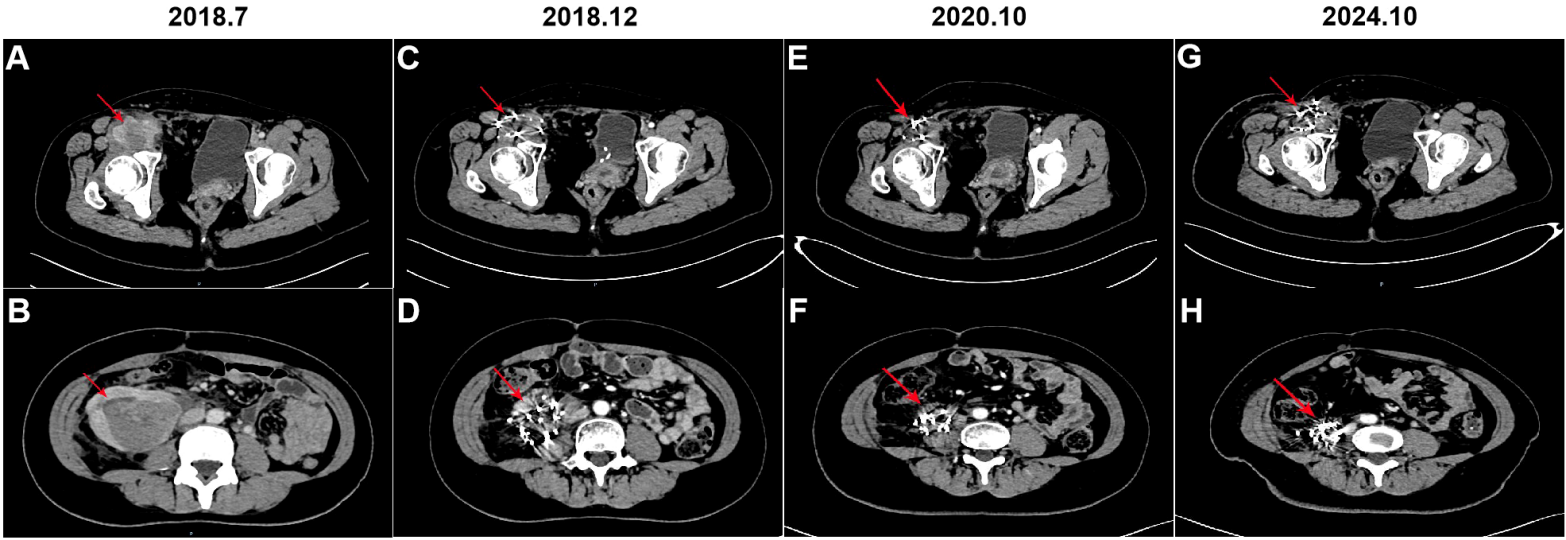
Computerized tomography (CT) was performed before and after iodine-125 seeds implantation therapy. (A, B) CT scan of the mass in the right groin and right abdomen, respectively (arrow); (C, D) CT scan of the mass in the right groin and right abdomen after 5 months of iodine-125 seeds implantation, respectively (arrow); (E, F) CT scan of the mass in the right groin and right abdomen after 3 years of iodine-125 seeds implantation, respectively (arrow); (G, H) CT scan of the mass in the right groin and right abdomen after 6 years of iodine-125 seeds implantation, respectively (arrow).
